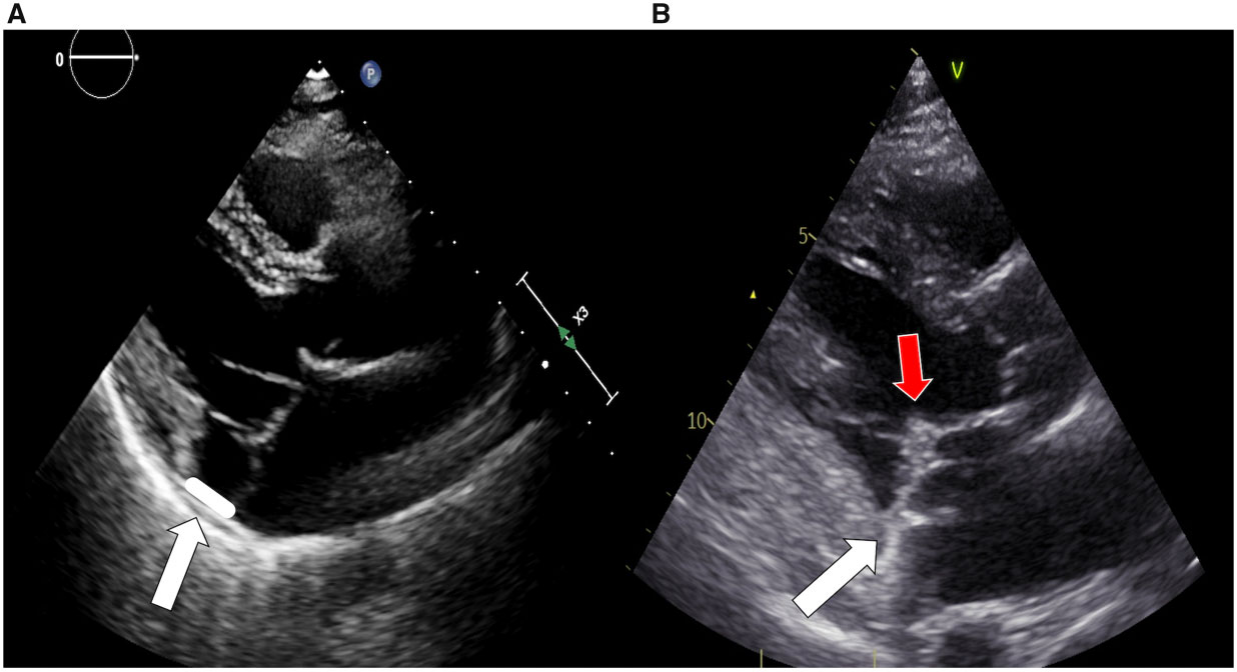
Parasternal long-axis view at transthoracic echocardiography of pre-operative and post-operative mitral valve. White arrows point at atrioventricular junction: pre-operative MAD (A) and its post-operative disappearance (B). Red arrow indicates the coaptation point, clearly below the annular plane.
